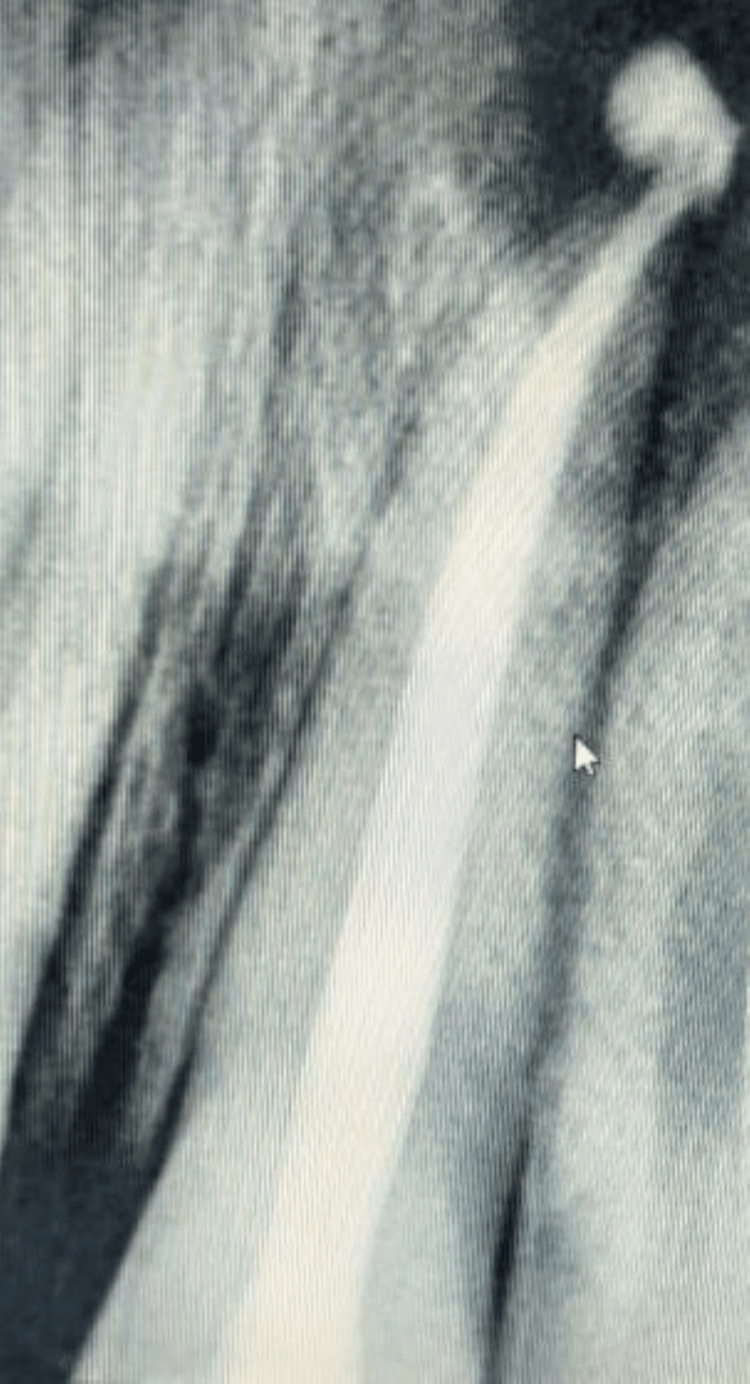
Post-obturation radiograph of tooth 21
